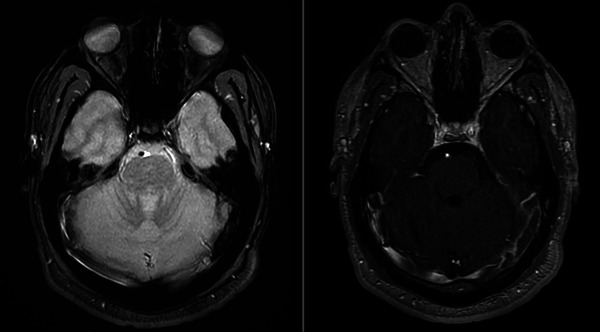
Brain MRI showing left lateral sinus thrombosis. (A) Axial T2* scan showing hypointensity in the left lateral sinus indicating the thrombus. (B) T1 with gadolinium showing an endoluminal defect in the left lateral sinus indicating thrombosis.
